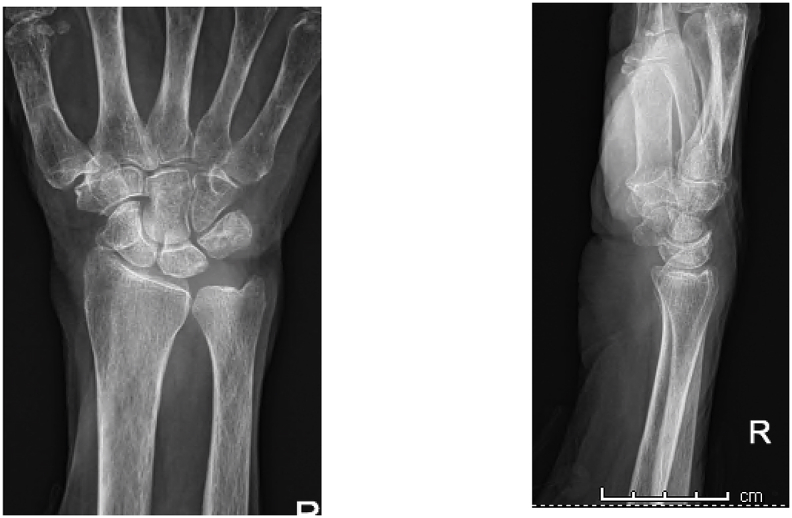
Anteroposterior and lateral radiographs views of the wrist showing no abbormalities.
